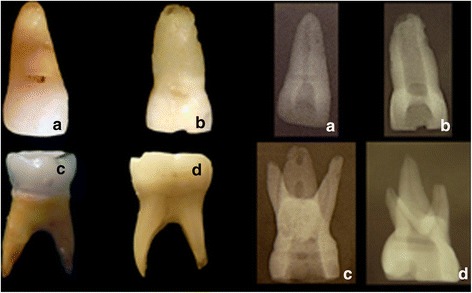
Visual and radiographic aspects of examples of artificial anterior (a) and posterior (c) primary teeth compared with natural anterior (b) and posterior (d) primary teeth
